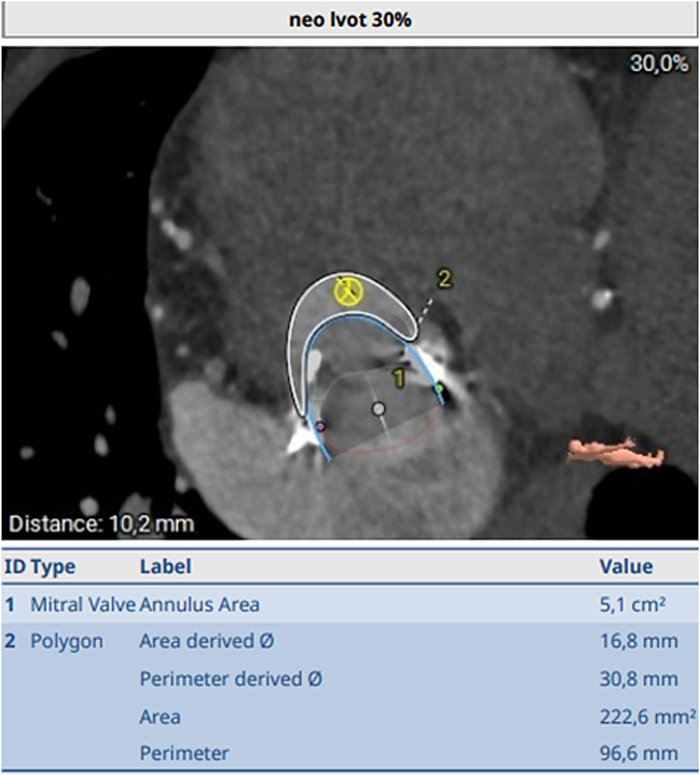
Computed Tomography Assessment of Neo-LVOT During Mavacamten Therapy Computed tomography–based measurement of the predicted neo-LVOT area during mavacamten therapy, measuring 222.6 mm^2^. LVOT = left ventricular outflow tract.
